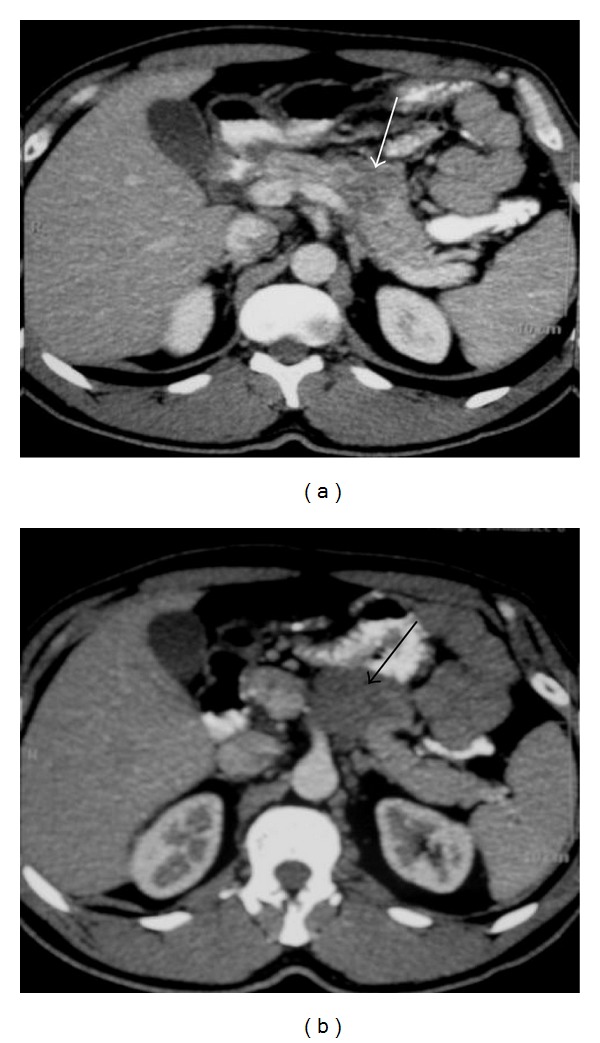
CT images of a 44-year-old man with pancreatic lymphoma. (a) Axial contrast-enhanced abdominal CT shows hypodense lesion involving the body of the pancreas (arrow). (b) Axial contrast-enhanced CT at a lower level demonstrates the lobulated hypodense mass (arrow).
